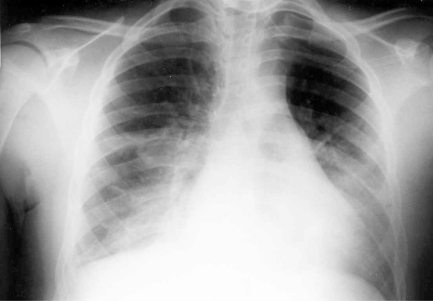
Chest X-ray of a patient in status asthmaticus who is Mycoplasma pneumoniae positive demonstrating bilateral basilar infiltrates.
